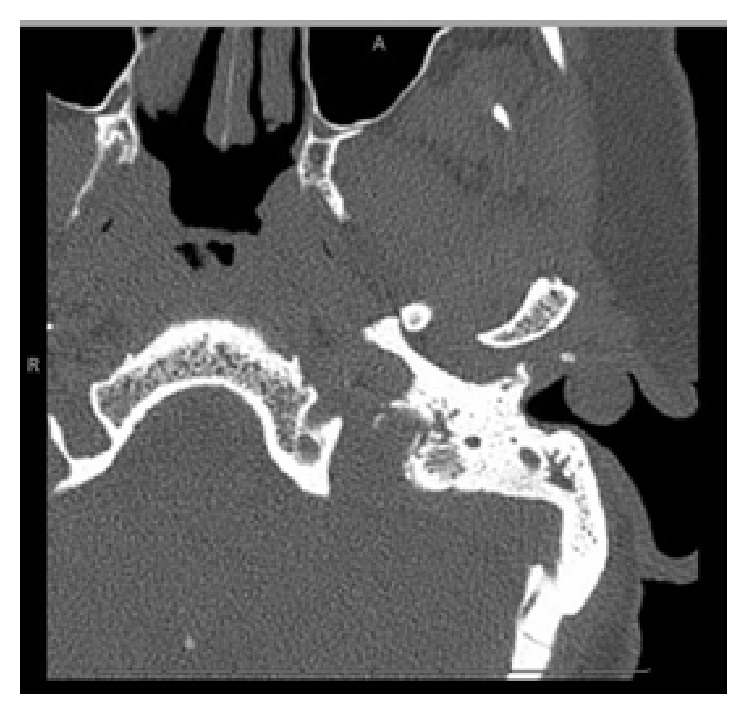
CT of the temporal bones shows a well-defined cystic lesion originating from the anterolateral portion of the left external auditory canal. The lesion has no deep extension, does not show any bone erosion, and does not involve the middle ear cleft.
